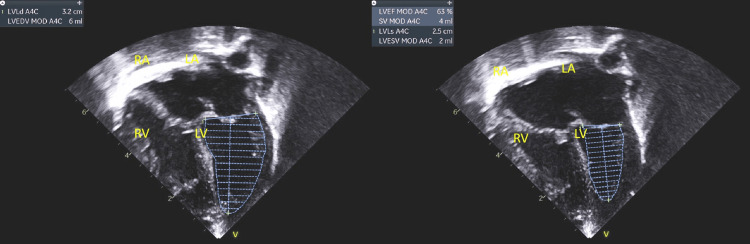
Apical 4 chamber view showing normal size LV with LVEF 65% done on 2nd day of life. LVEF: Left ventricle ejection fraction
